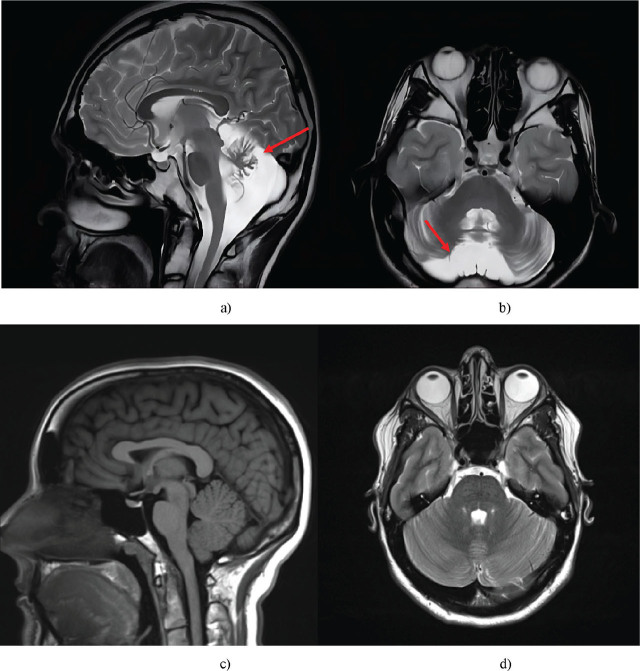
Brain MRI of the eldest child (Patient 1): a) sagittal; b) axial sections. Healthy brain: c) sagittal; d) axial slices. The pathological features observed include severe cerebellar vermian atrophy, compensatory enlargement of the posterior cerebellar cistern, and marked decrease in overall cerebellar volume, with thinning of the cerebellar folia and deepening of the cerebellar sulci. *Source*: a-b were obtained during the study and c-d are reproduced from [34].
